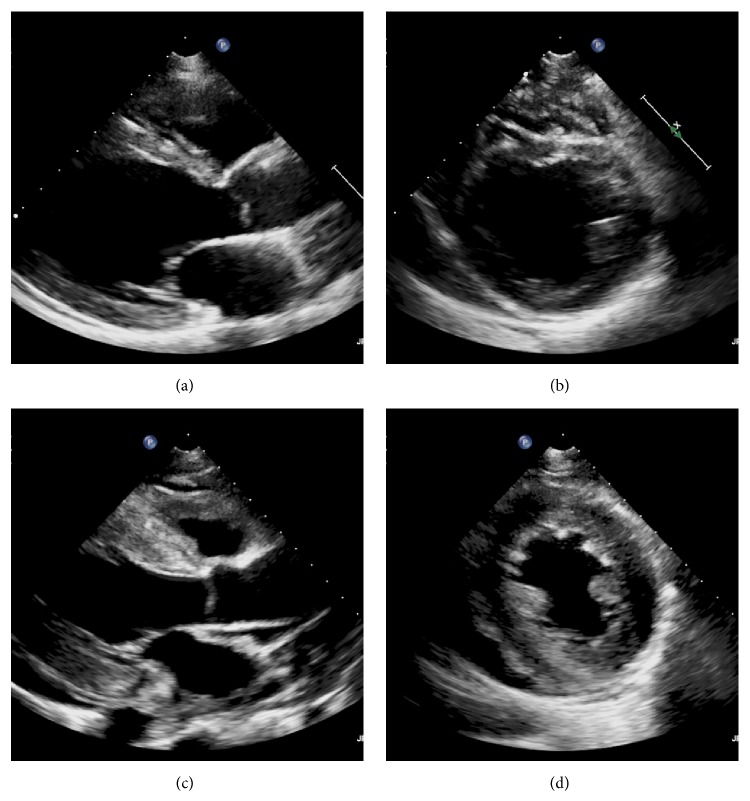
Telediastolic parasternal long (panel (a)) and short (panel (b)) axis view showing normal thickness of the myocardium 2 months before admission. Panels (c) and (d) show telediastolic parasternal long and short axis view, respectively, of the same patient, 6 days after admission. Impressive diffuse myocardium thickening is observed.
